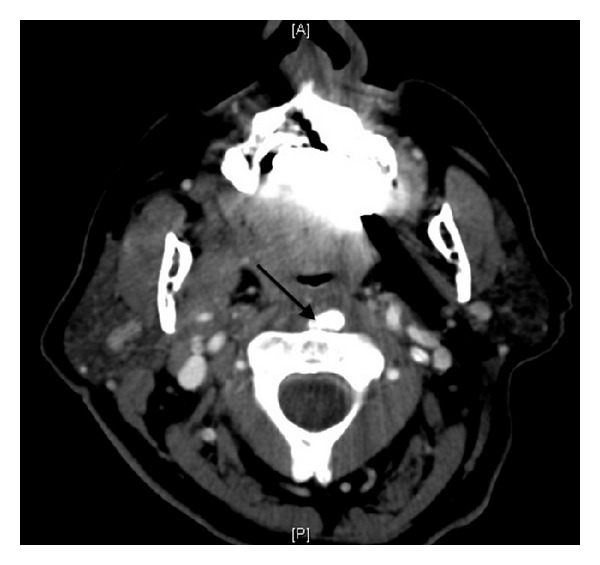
Axial CT of the neck with IV contrast shows calcifications anterior to the body of C2 (arrow).
